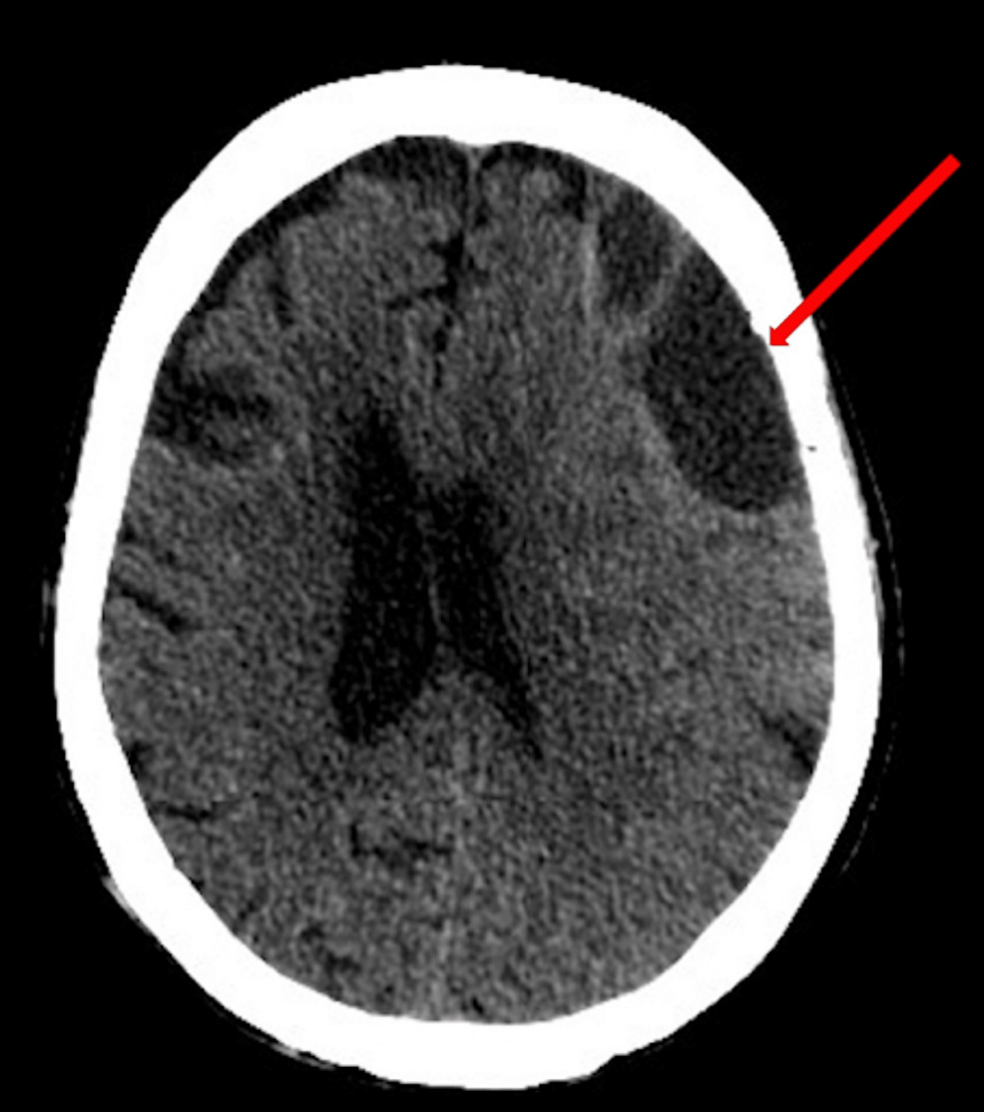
Initial CT scan displaying the 130 ml hematoma with concurrent 7 mm of midline shift
